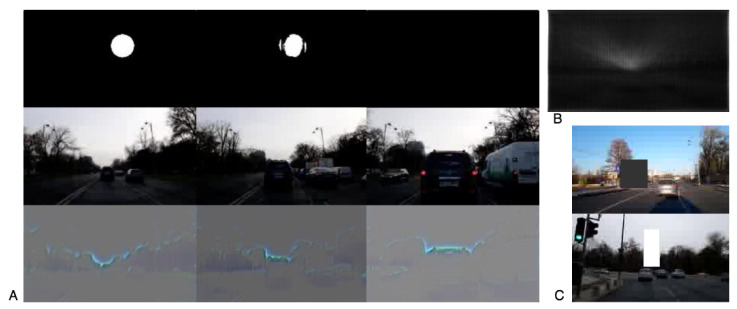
Analyzing the network’s attention by applying the guided backpropagation technique. (A) The top row shows the prediction of the network for the observer’s location, while the bottom one shows the visual cues of most interest for the network. It can be seen that as the camera approaches the SUV vehicle, the skyline shape changes and the segmentation of the dot fades away. (B) The guided backpropagation mean pixel activation values over the training set images. (C) Augmented images (with occlusions being added in the form of rectangles of constant grey level) used for robust training.
